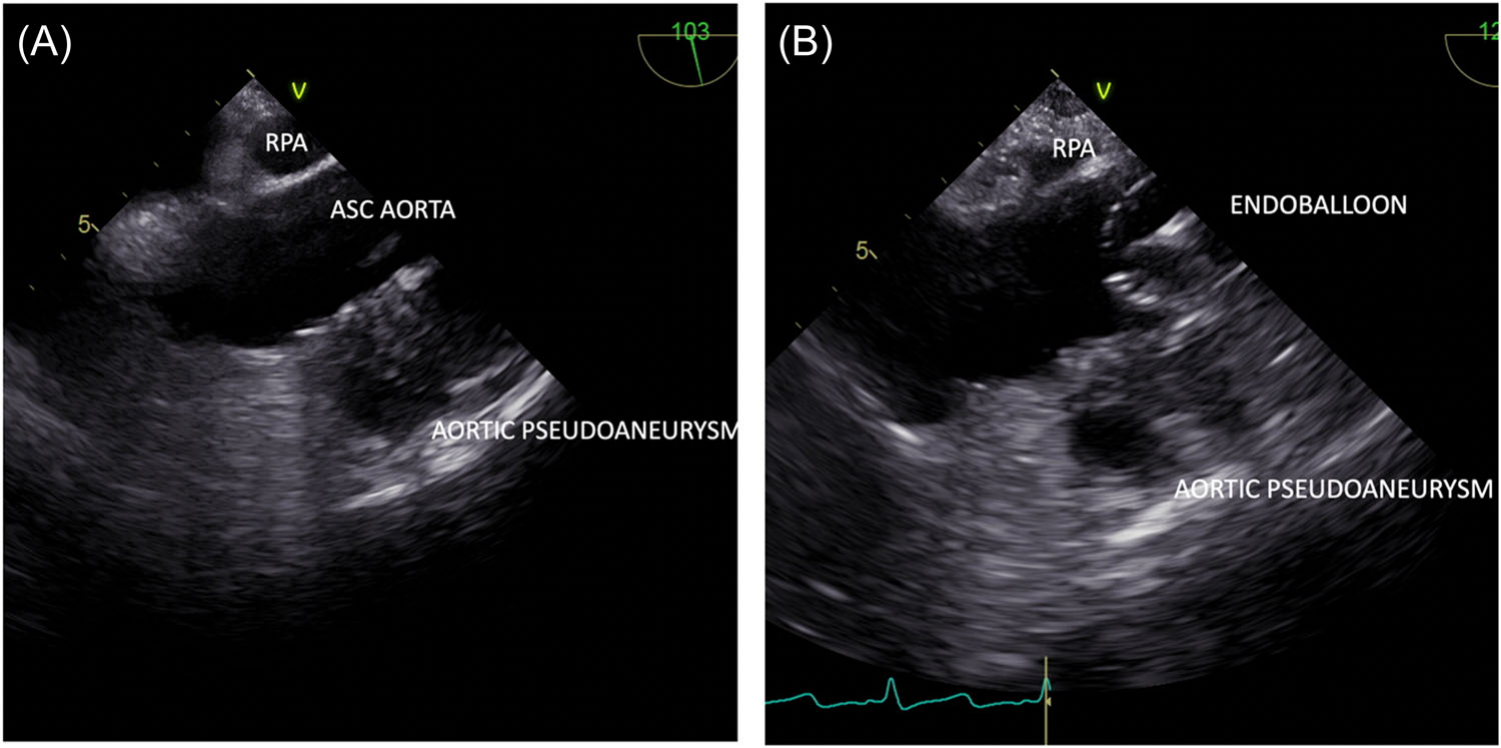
(A) TEE image demonstrating preoperative aortic pseudoaneurysm along the anterior aspect of the ascending aorta, the right main pulmonary artery was utilized as a landmark for endoballoon positioning safely above the patent proximal coronary anastomoses (B) Intraoperative image demonstrating the inflated endoballoon in situ above the patent grafts while cardioplegia was being delivered to the aortic root. TEE, transthoracic echocardiogram
